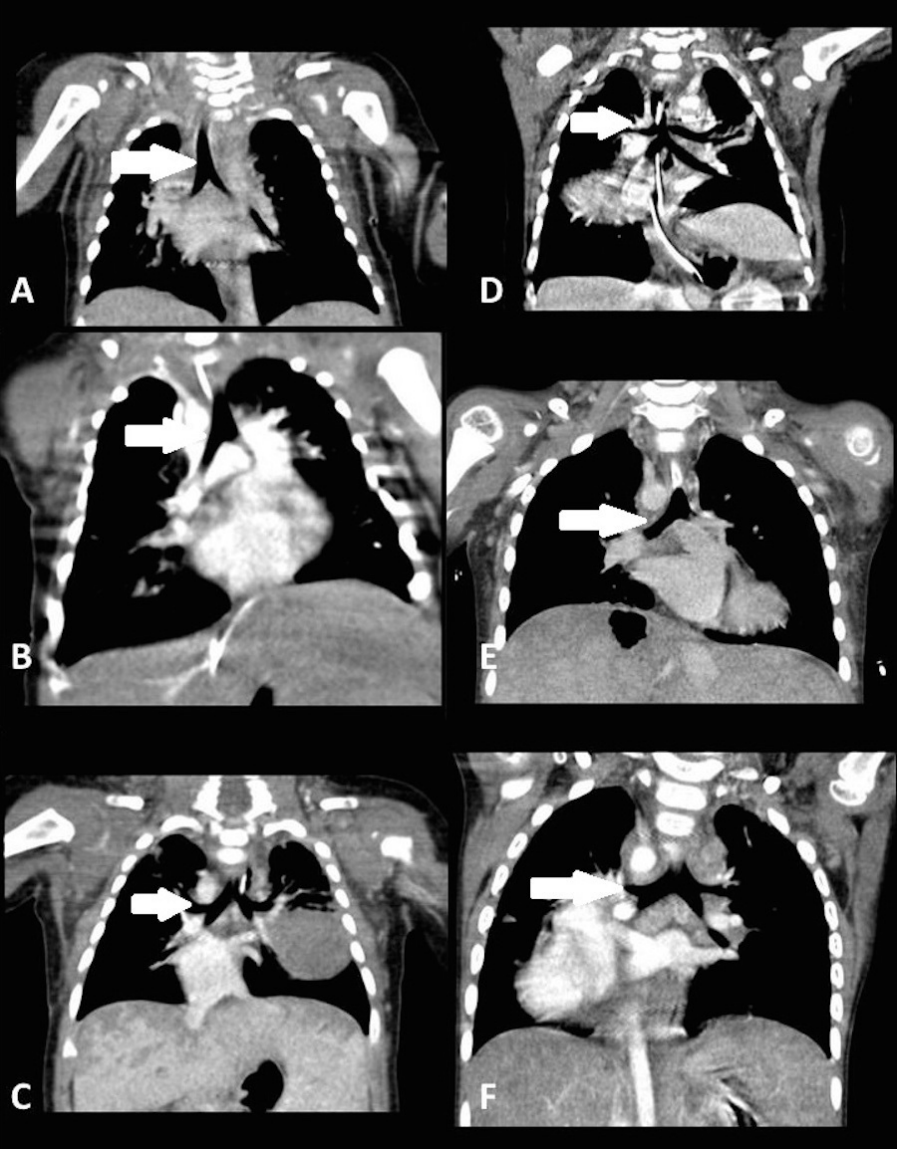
Bronchial isomerism Computed tomography of the chest in the coronal plane from six different patients demonstrating bronchial isomerism. Panels A, B, and C demonstrate bronchial angles consistent with right-sided bronchial isomerism while panels D, E, and F demonstrate bronchial angles consistent with left-sided bronchial isomerism. Arrows point to the bronchial tree.
